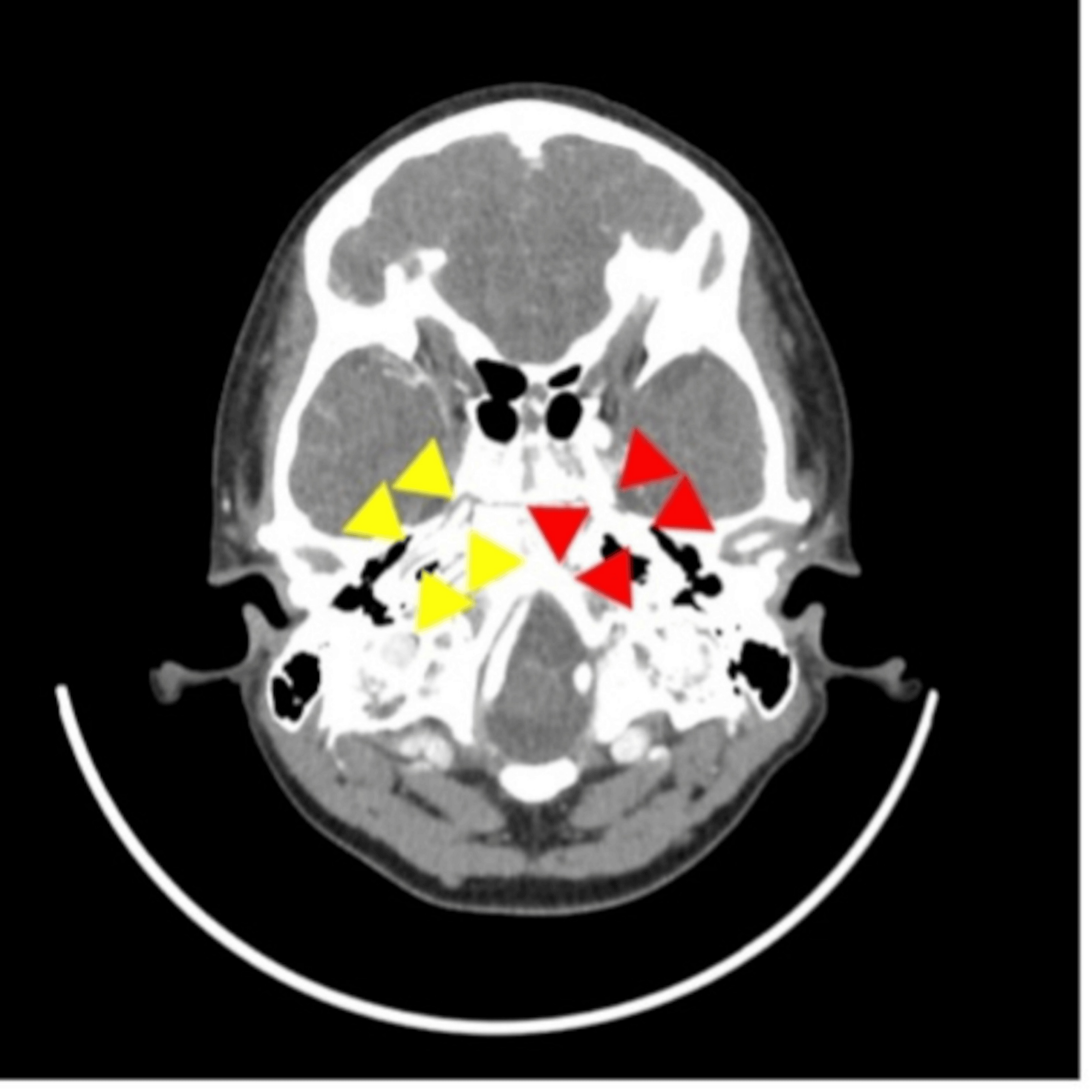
Contrast-enhanced computed tomography of the head on day 12 of admission. Contrast-enhanced computed tomography of the head on day 12 of admission shows circumferential thickening of the vessel wall of the right middle cerebral artery (yellow arrowheads). There is no contralateral vessel wall thickening (red arrowheads).
